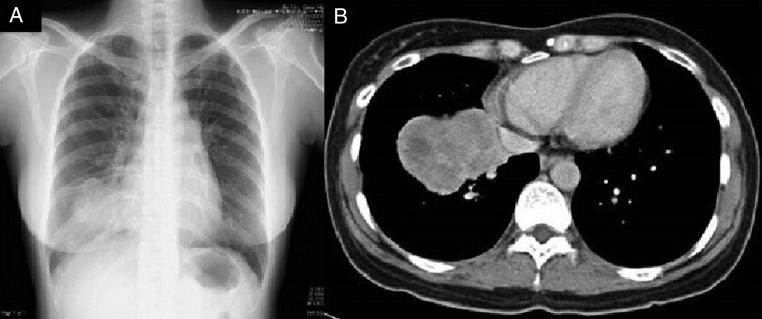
(A) Chest radiograph shows a mass in the right lower lung field. (B) Chest CT shows ∼70 mm mass in the right lower lobe. The border of the mass is irregular, and the interior is heterogeneous.
